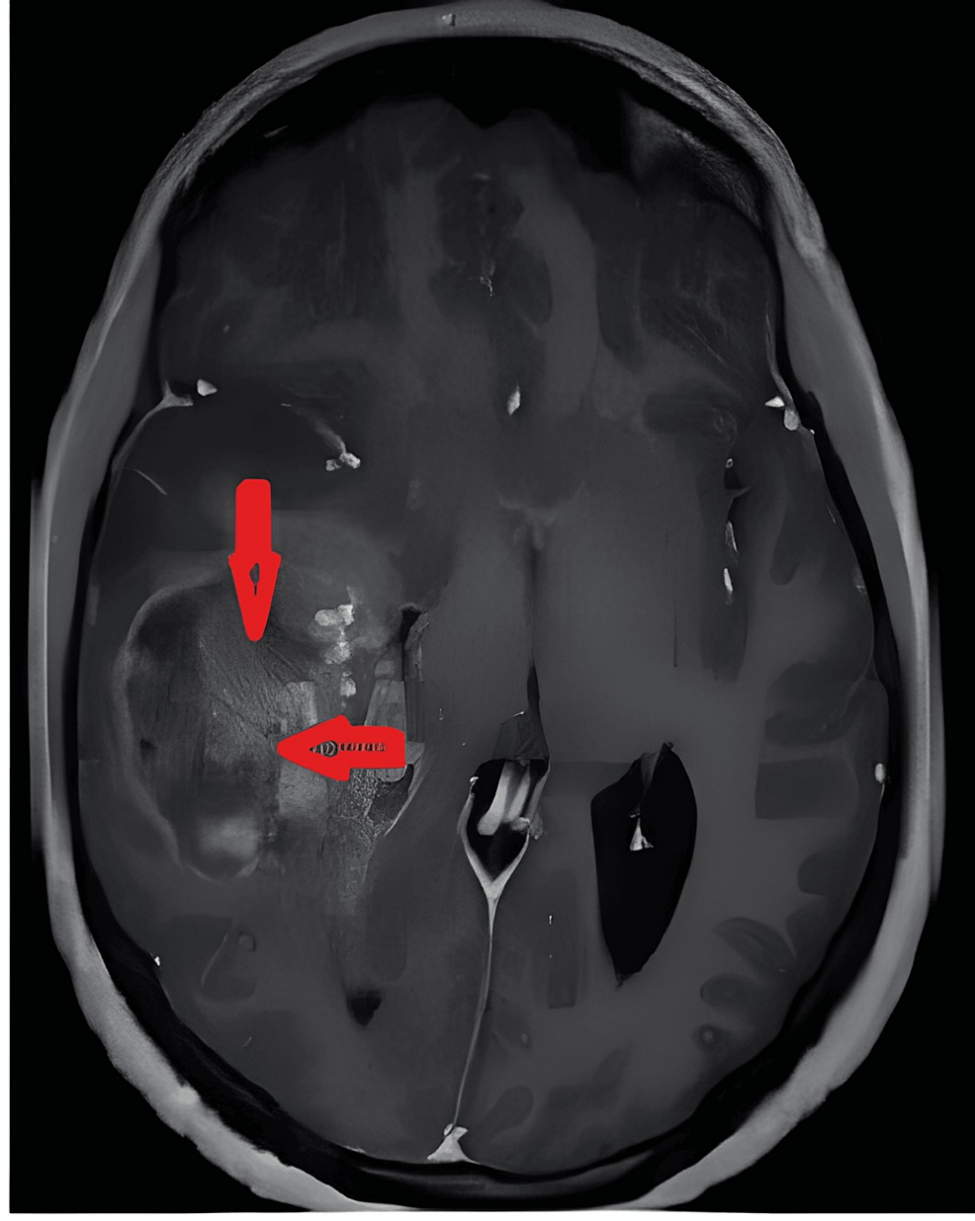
Brain MRI (post-steroid therapy). Treatment MRI (post-steroid therapy) of an 11-year-old girl with right temporal glioblastoma multiforme with heterogeneous enhancement in axial sagittal T1-weighted imaging with contrast agent. The tumor mass had caused a midline shift and showed central enhancement.
